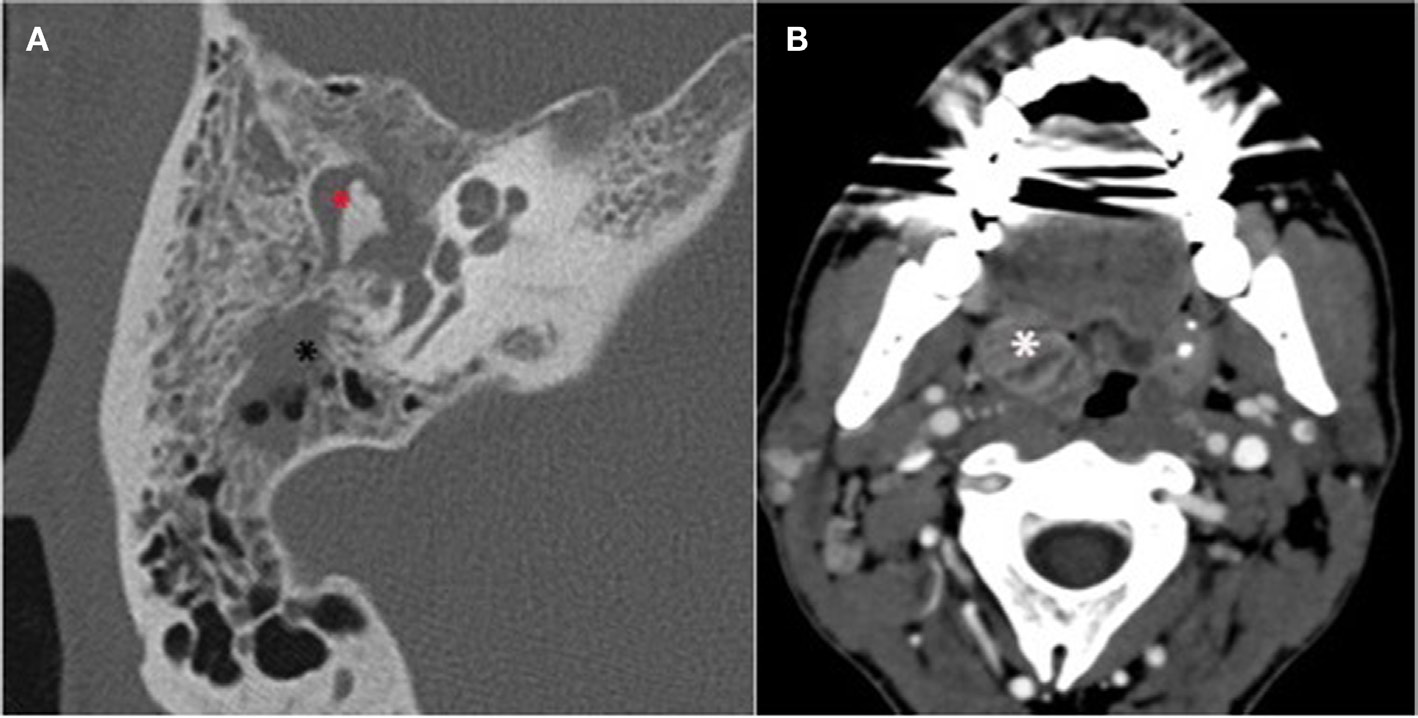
Mastoid CT scan of patient 3. (A) High-resolution axial section focus of the right temporal bone. Acute otitis media with tympanomastoid homogenous opacity inflammatory filling of the whole tympanic cavity (
